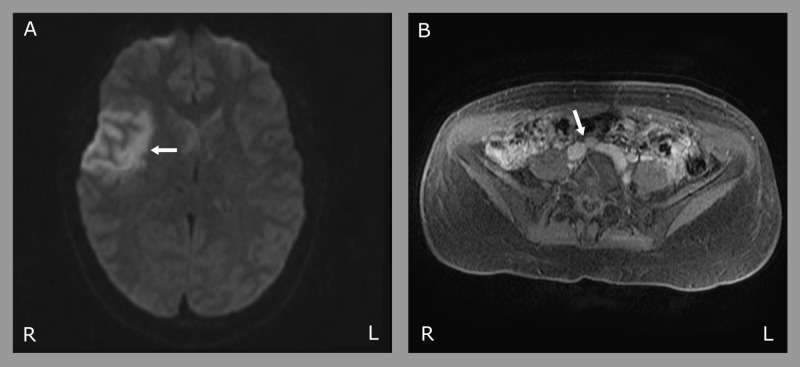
MR imaging. (A) Axial diffusion weighted imaging (DWI) MRI showing a subacute right frontal opercular infarct. (B) Axial pelvis magnetic resonance venography (MRV) showing compression of the left common iliac vein by the overlying right common iliac artery and the posterior vertebral body consistent with May-Thurner syndrome.
